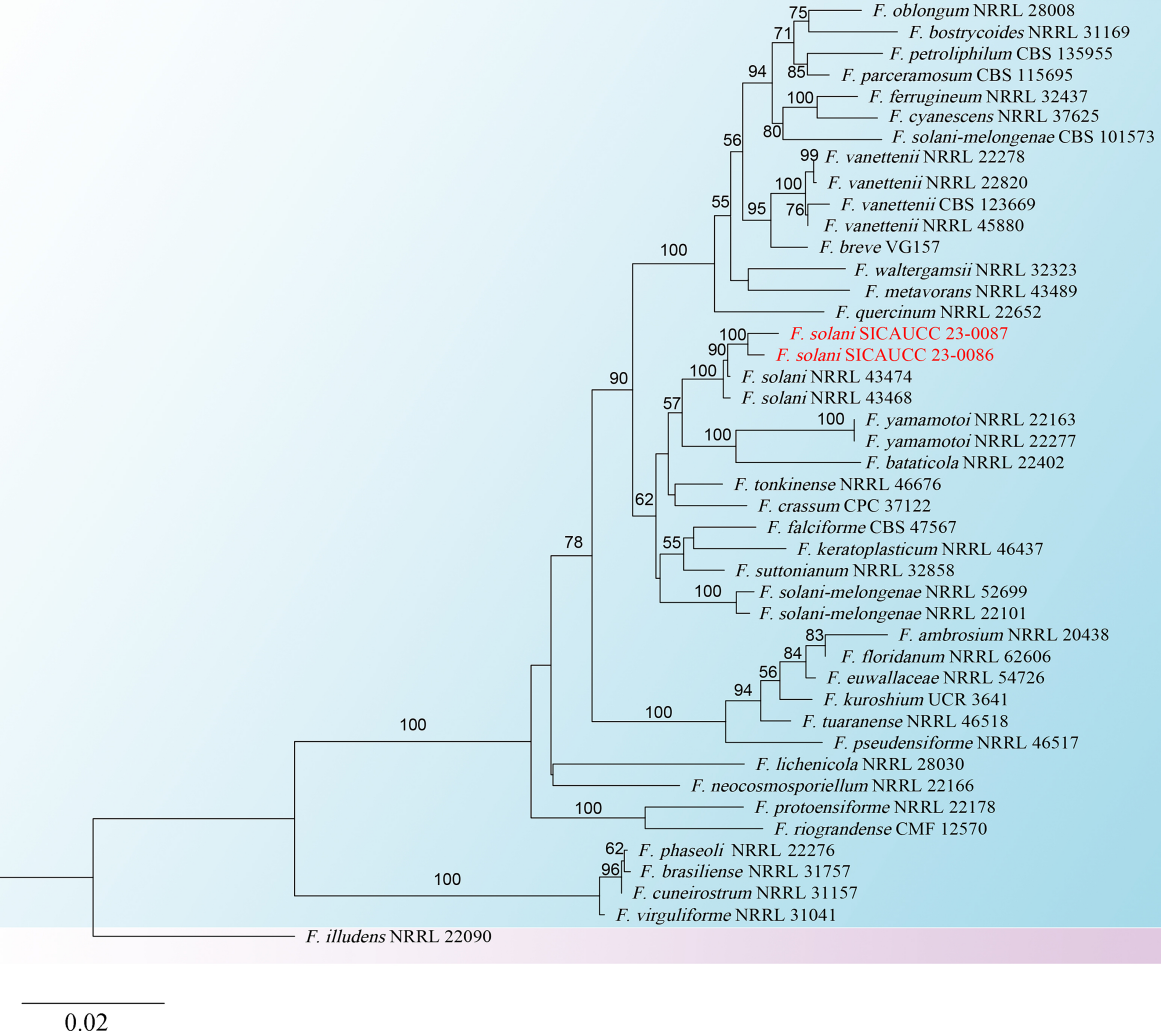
Phylogram generated from RAxML analysis, based on combined ITS, *rpb*2, and *tef*1-α sequence data of isolates. The best-scoring RAxML tree with a final likelihood value of −13876.220691 for ITS, *rpb*2, and *tef*1-α sequence data. The tree is rooted with *F. illudens* (NRRL 22090). The matrix had 1,020 distinct alignment patterns with 29.25% undetermined characters and gaps. Estimated base frequencies were as follows; A = 0.240624, C = 0.283489, G = 0.253665, T = 0.222222; substitution rates AC = 1.815518, AG = 5.297331, AT = 2.169463, CG = 1.145656, CT = 12.381417, GT = 1.000000; gamma distribution shape parameter *α* = 0.189753. Species identified in this study are indicated in red. Bootstrap support values (over 50%) from maximum likelihood are given at the nodes.
